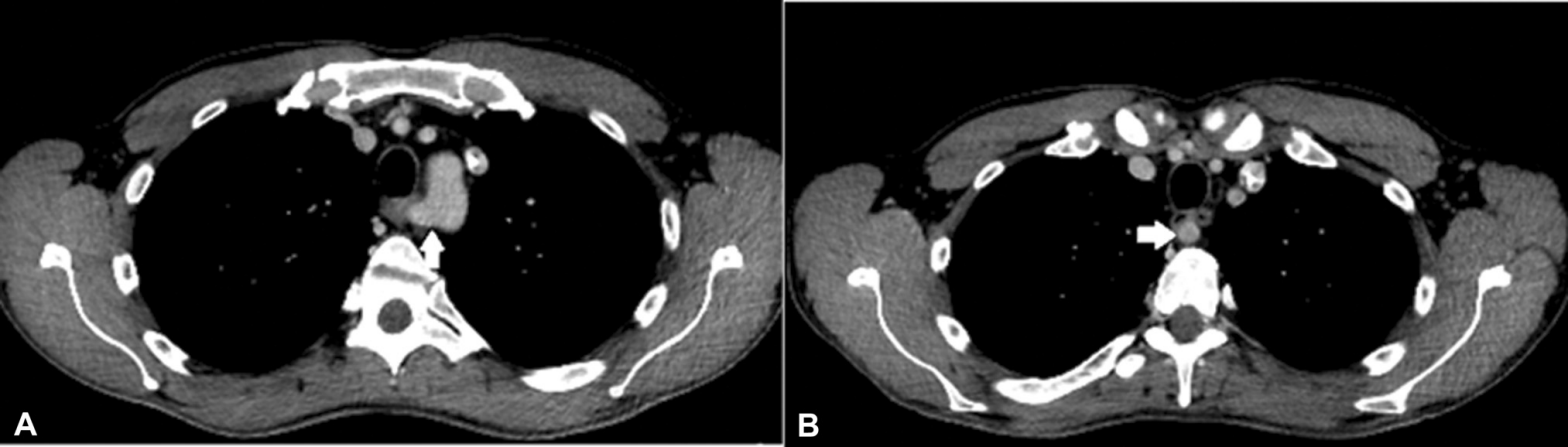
Computed tomography scan: axial oblique views demonstrating the origin of the right aberrant subclavian artery directly from the aortic arch (A) and its relation to the esophagus (B).
